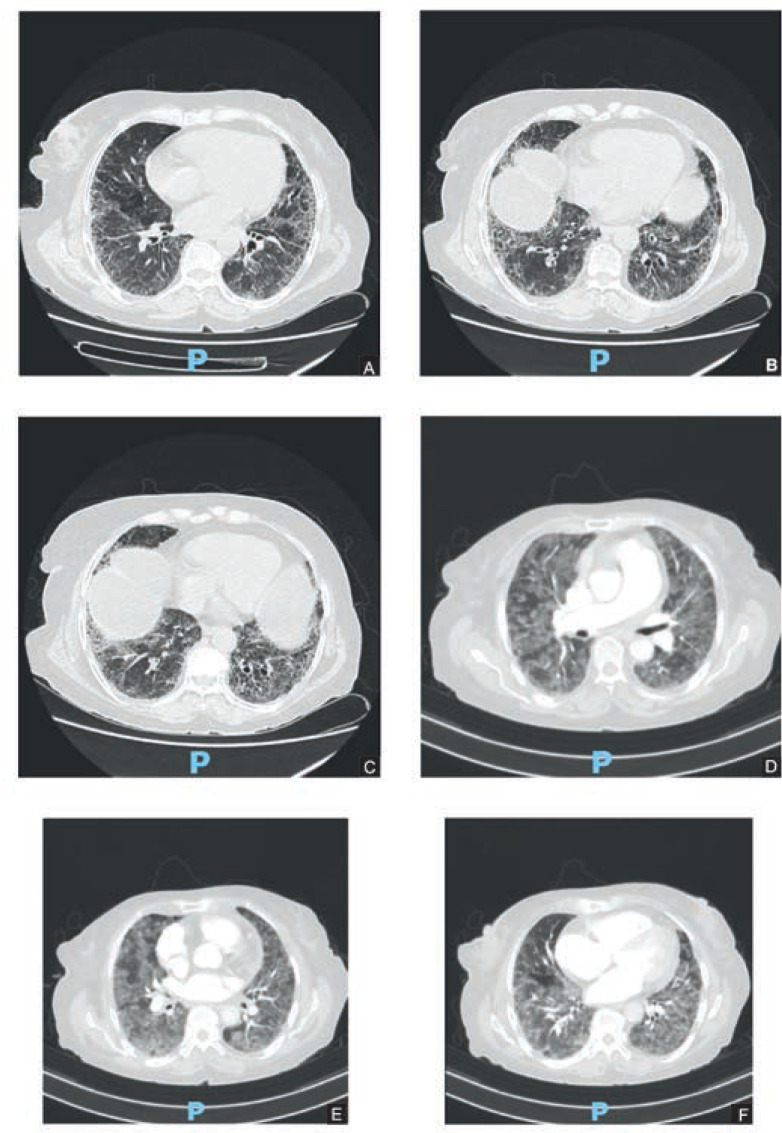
High-definition chest tomography (HRCT). (A–C) Increase in the peribronchovascular interstitial thickening interstice, thickening of the interlobular septum and honeycomb transformation, mostly in the mid and basal pulmonary fields, as well, bronchiolectasis caused by traction, suggesting usual interstitial pneumonia (UIP), along with mild cardiomegaly and increased diameter of the pulmonary trunk, reaching 37 mm. (D–F) HRCT taken after the deterioration of the patient, showing ground glass opacities along with interlobar septal thickening. In these boxes, there are no changes in the vascular portions or in the cardiomegaly measurement.
